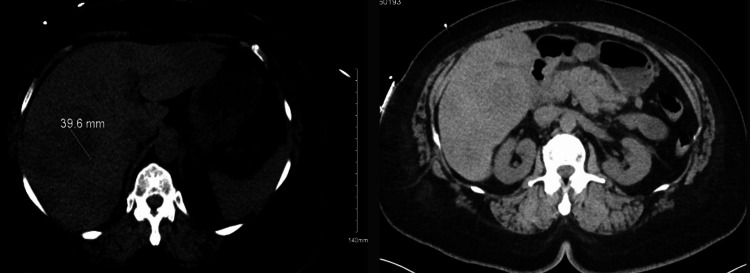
CT Abdomen Pelvis Extensive liver masses suspicious for metastatic disease and suggested enlargement of the pancreatic body, which may reflect a neoplasm or metastasis.
